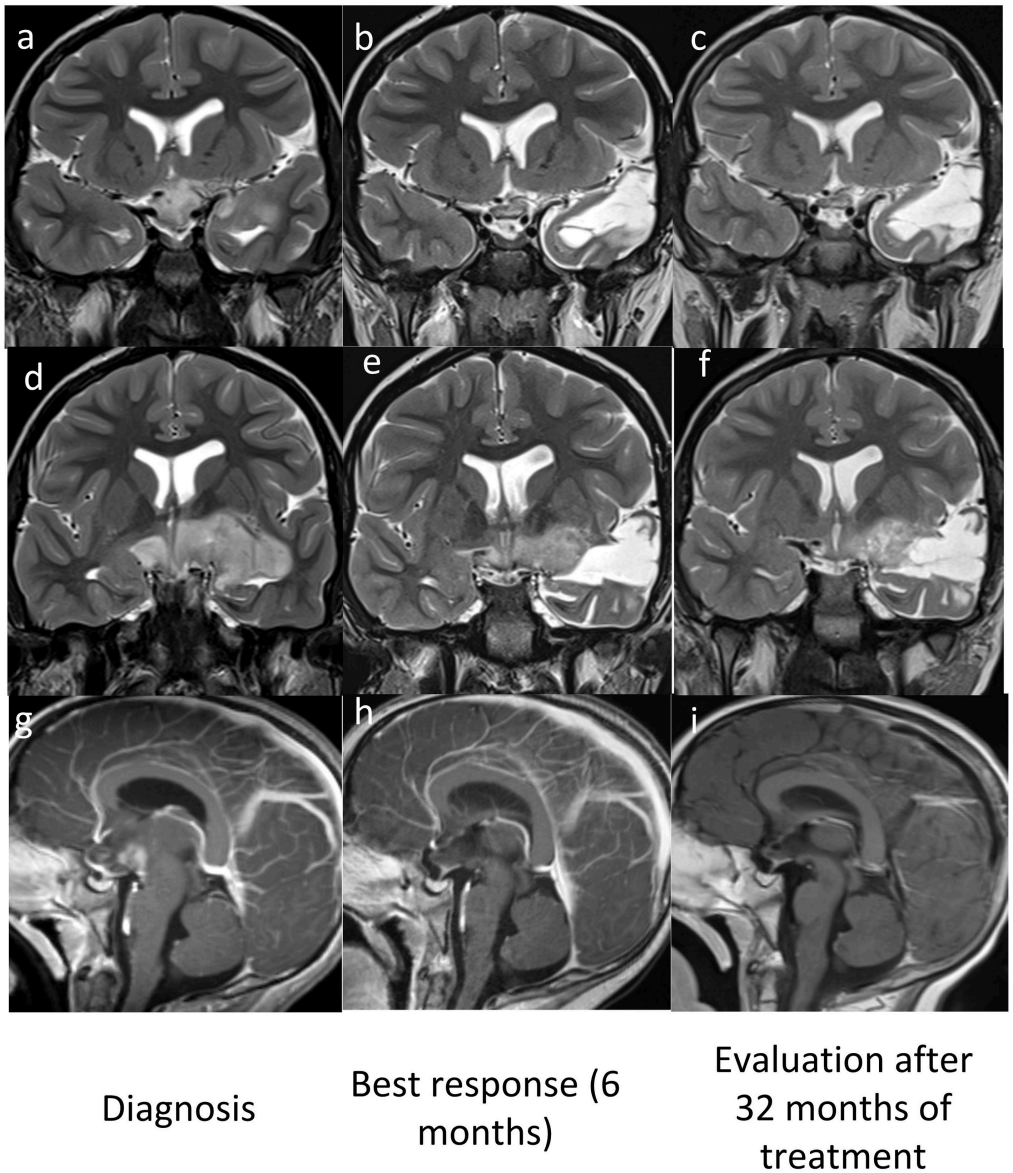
MRI pre (a,d,g) and post Vemurafenib (b,c,e,f,h,i) coronal T2w (a–f) and sagittal Gd T1w (g–i) images show best response after 6 months (b,e,h) and stable disease after 32 months (c,f,i).
